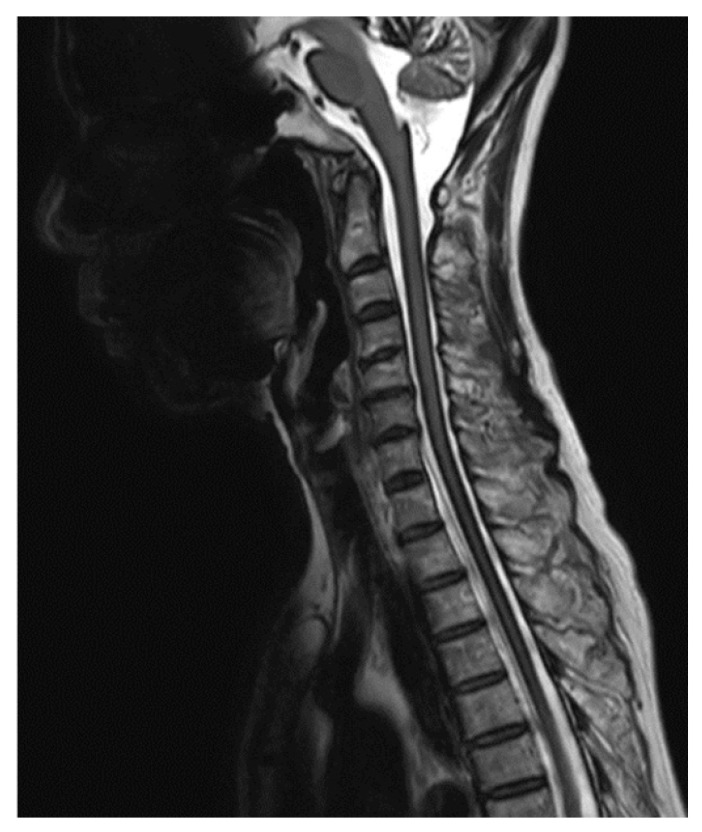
T2-weighted magnetic resonance imaging (MRI) of the cervical to upper thoracic spine reveals hypointensity in the superficial areas of the central nervous system which is characteristic of superficial siderosis. An anterior epidural fluid collection spanning the C7 to T10 levels is also visible.
